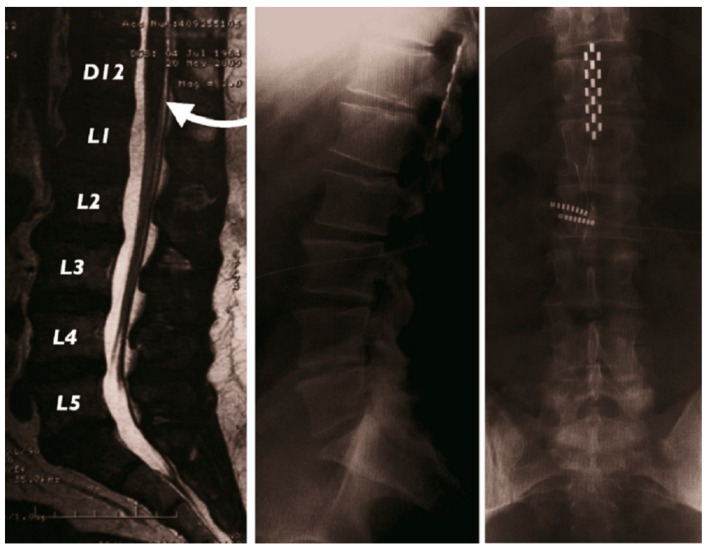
Pre-operative MRI showing the projection of the conus medullaris at T12-L1 vertebral level (on the left) and post-operative X-ray localizing the lead position, at the same vertebral projection (in the middle and on the right)/from Rigoard et al. [32] with permission.
